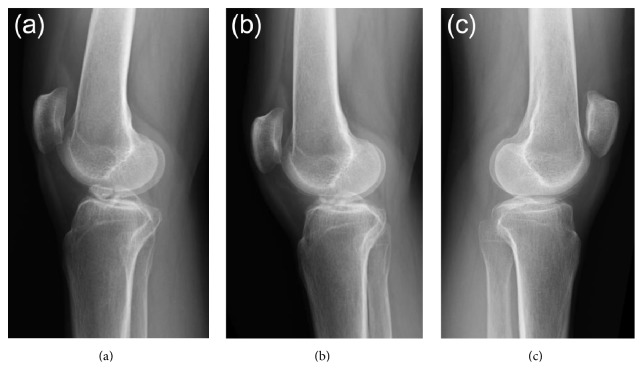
Plain radiography of our patient. (a) Lateral view of the right knee joint on first examination at our hospital, showing complete displacement of the avulsed fragment at the right anterior tibial eminence from the tibial bone mass and impingement of the fragment on the femoral intercondylar notch during knee extension. (b) Lateral view of the right knee joint 2 years after resection of the anterior region of the avulsed fragment at the anterior tibial eminence, showing no impingement of the avulsed fragment on the femoral intercondylar notch during knee extension. (c) Lateral view of the left knee joint at the time of initial examination at our hospital.
